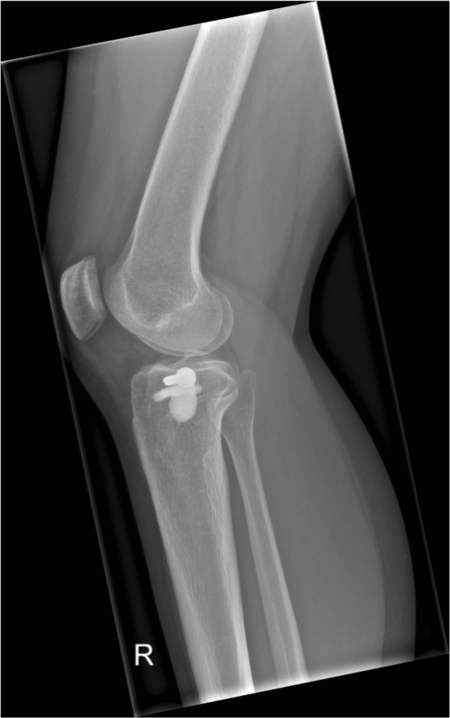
Lateral X-ray view of a successful cement-augmented tibioplasty of a tibial plateau fracture (Schatzker II)
